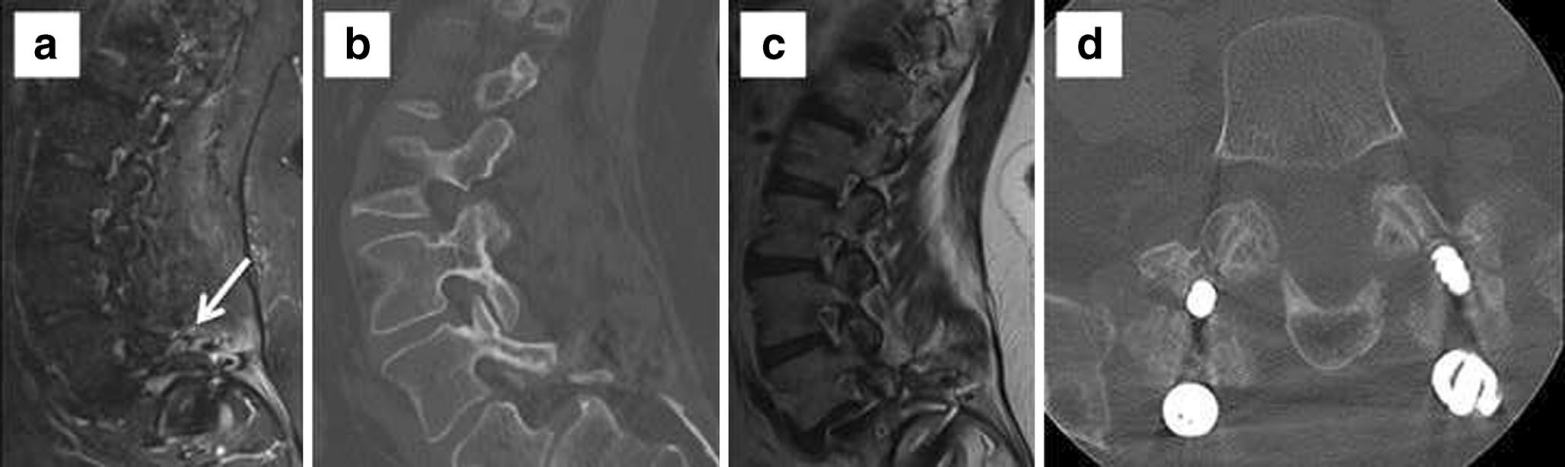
Pseudoarthrosis with lack of bony fusion on CT (b and d) and persistent increased signal on STIR and T2 WI (a and c) with worsening anterolisthesis
